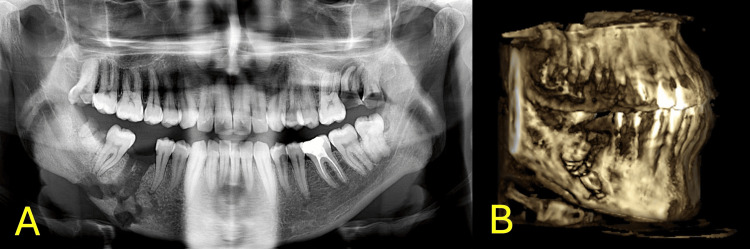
Radiographic examination images A. Pre-operative panoramic radiograph. B. Pre-operative 3-dimensional computed tomography.
